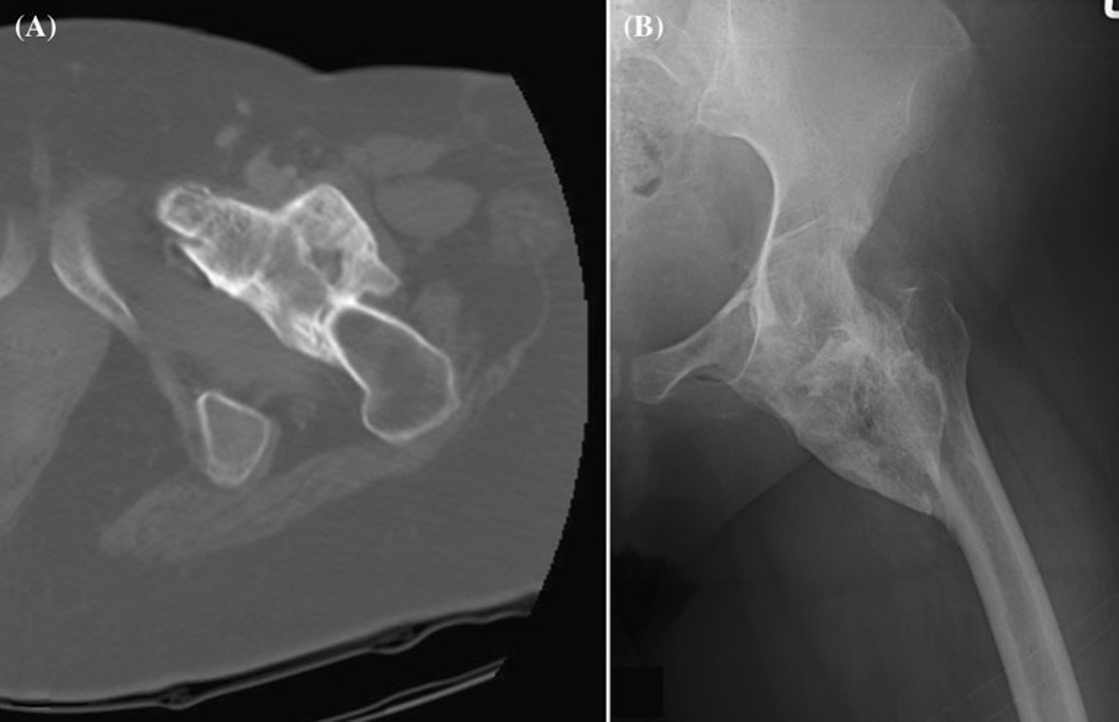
a Axial computed tomography scan and b anteroposterior radiograph of the left hip of a 49-year-old woman with anteromedial neurogenic HO of the hip after spinal cord injury (patient 10; type 3a)
